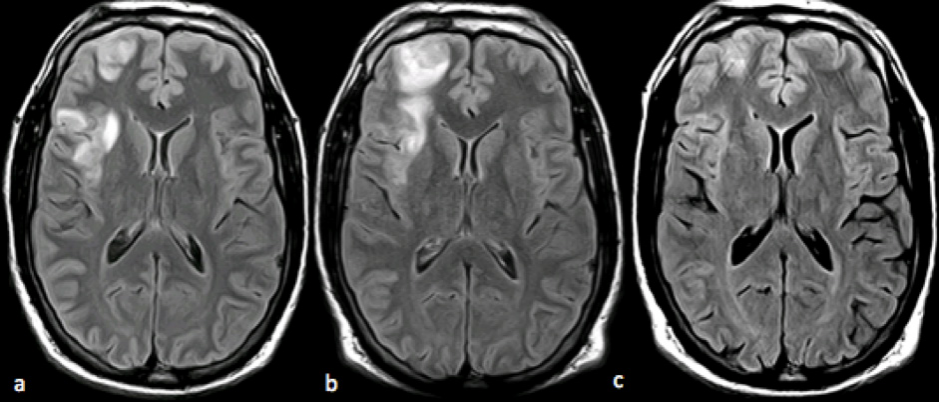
Head MRI axial FLAIR; before treatment (initial MRI, (a); 15 days later, but before initiation of specific treatment (b): showing lesions increased in size with deep white matter involvement and increased mass effect; three months after treatment (c), showing very small residual foci. FLAIR, fluid-attenuated inversion-recovery.
